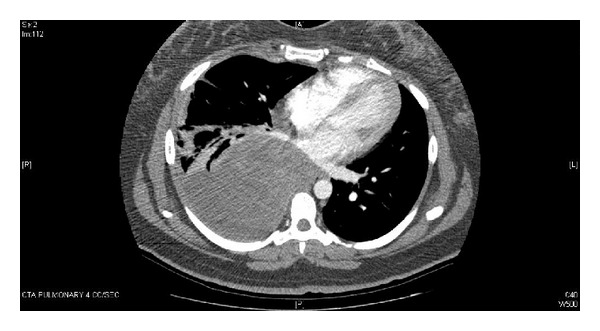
Helical CT with contrast demonstrating a large partially calcified mass within right hemithorax with broad-base apposition to mediastinum (24DEC2009).
